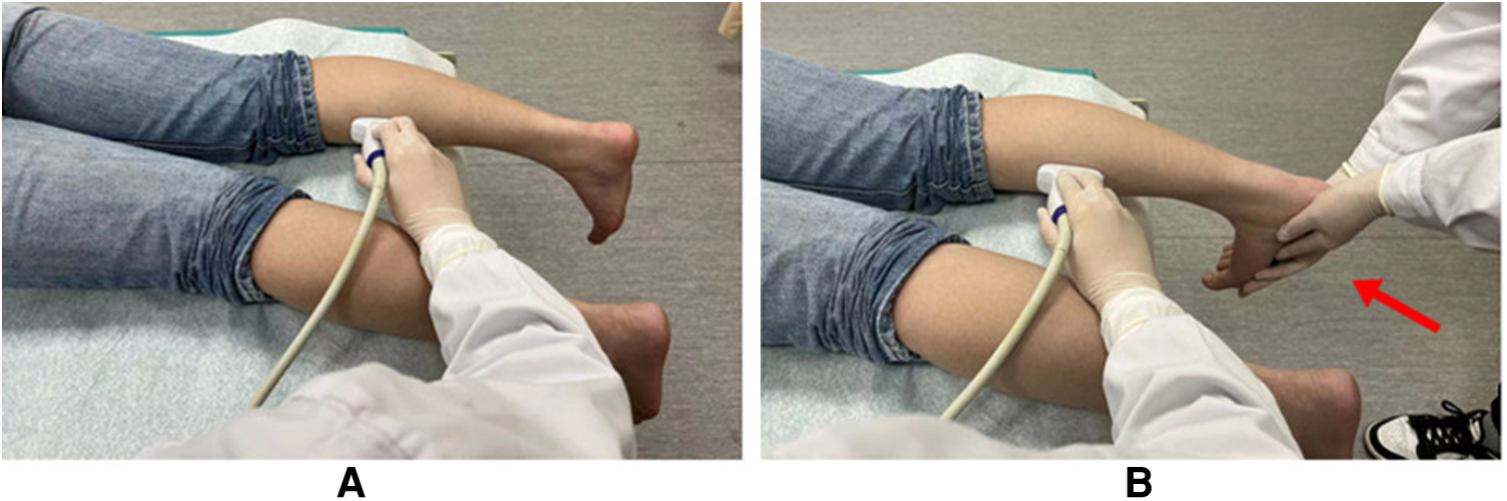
Photos of the rest position (A) and contraction position (B) under multimodal ultrasound examination. The extension of the foot's dorsum under the contraction position is indicated by the red arrow.
